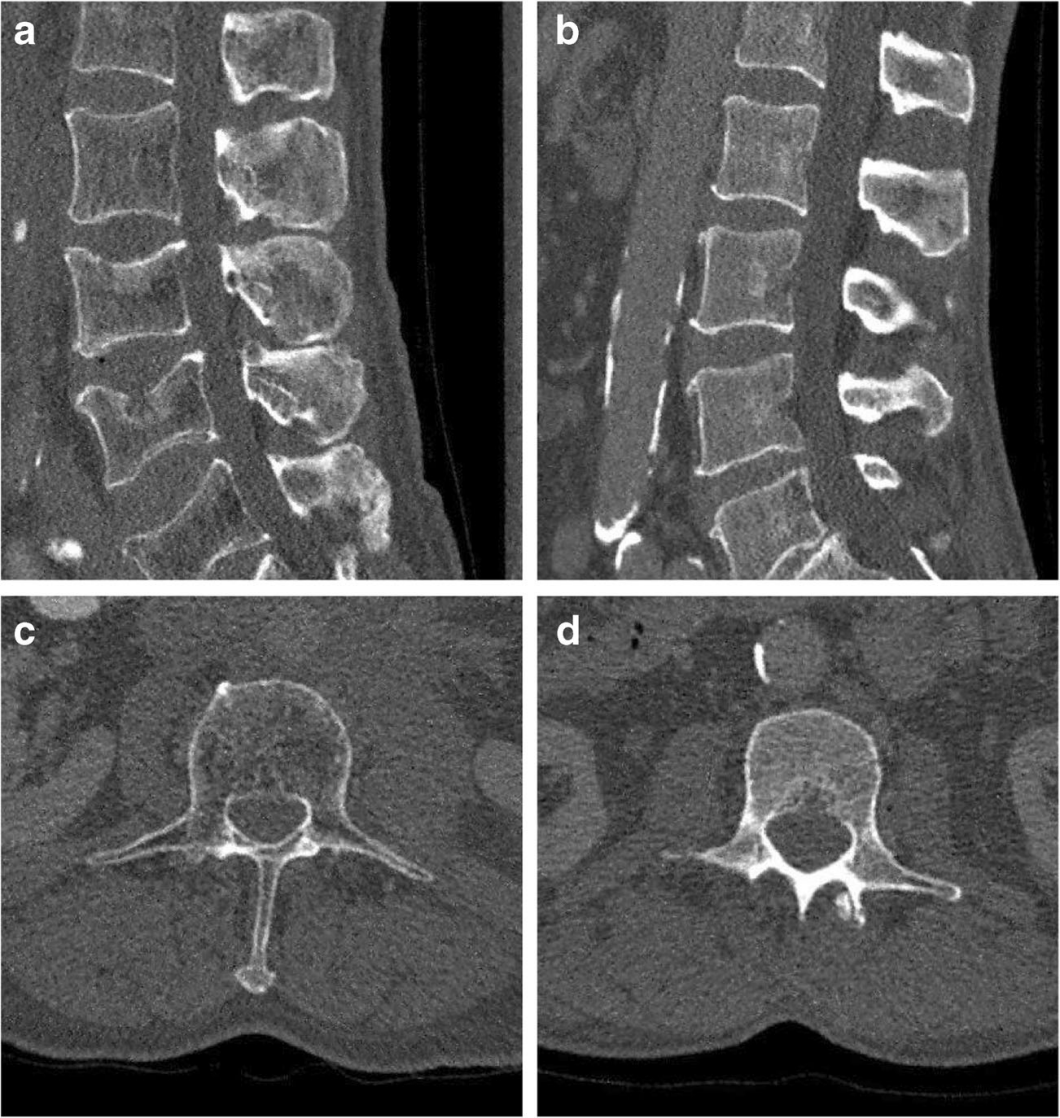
Representative reconstructions of the lumbar spine (L1–L5) of in-vivo spine multidetector CT (MDCT) data at the original dose. The left column depicts a subject with a fracture; the right column displays the matched healthy subject with regard to age and gender. The original dose was at 120 kV, 107 mAs (left) and 114 mAs (right) (exact tube current was modulated). Window level was 300 HU and width was 1,500 HU. Field of view was 180 × 153 mm^2^ for (a) and (b), and 156 × 156 mm^2^ for (c) and (d)
